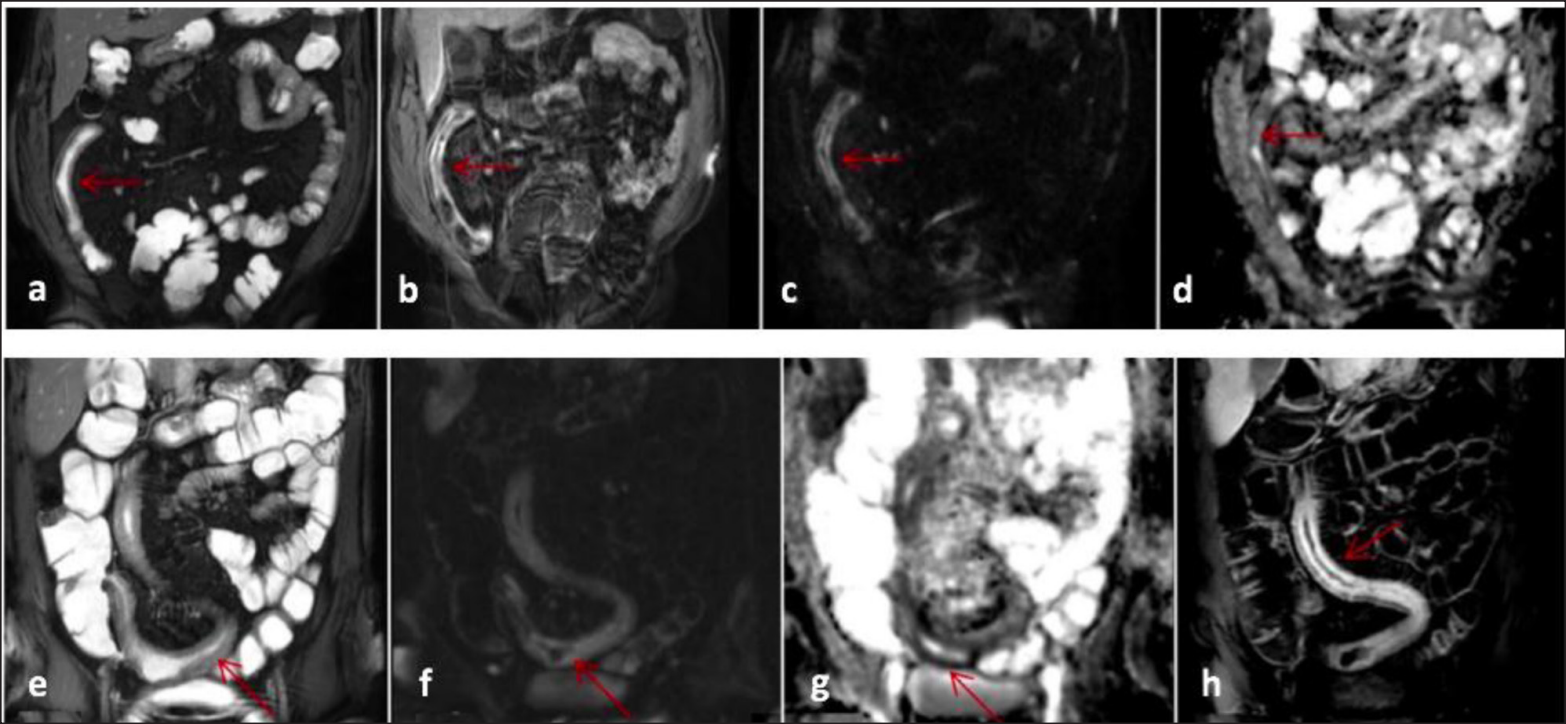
MR images of a patient with Crohn’s disease involving distal ileum (a–e) and another patient with involvement of a long segment of distal ileum and ileocecal junction (e–h). The images of the first patient show wall thickening and mural T2 hyperintensity on coronal FIESTA image (a), contrast enhancement along thickened ileal wall (b), mural diffusion restriction as suggested by DWI hyperintensity (c) with corresponding signal decrease along thickened ileal wall on ADC mapping (d). The images of the second patient show diffuse mural T2 signal increase with ileal wall thickening on coronal FIESTA image (e), mural DWI hyperintensity along involved ileal segment (f) with corresponding mural hypointensity on ADC mapping consistent with restricted diffusion (g) and mural contrast enhancement after intravenous gadolinium (h).
